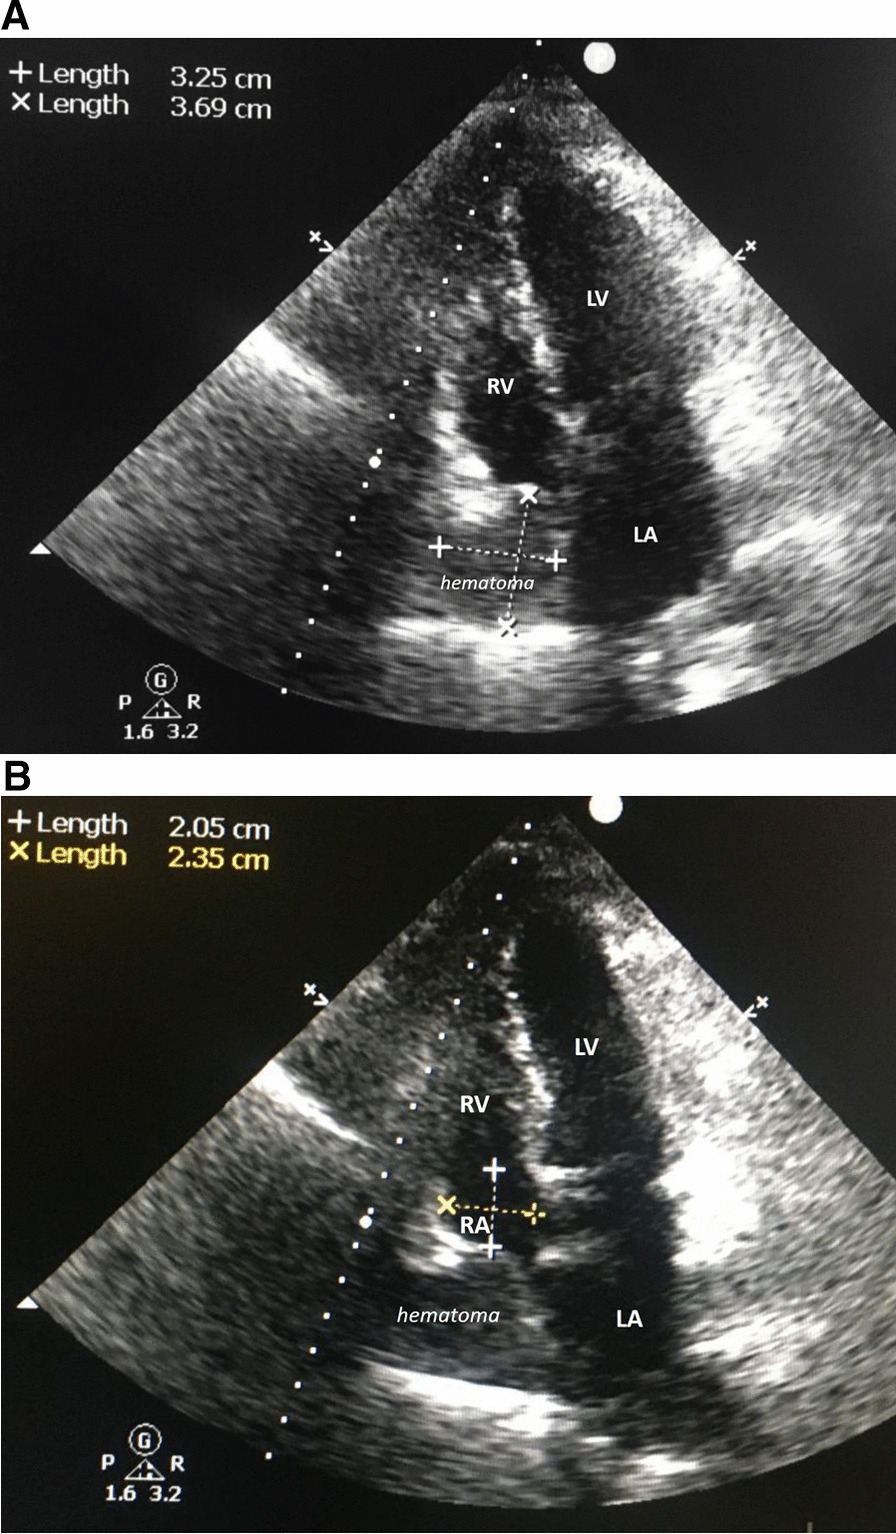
A Transthoracic echocardiography with apical four-chamber view, showing a round shaped 32 × 37 mm hematoma compressing the right atrium (systolic view). *LV* left ventricle, *RV* right ventricle, *LA* left atrium. B Transthoracic echocardiography with apical four-chamber view (after minor probe tilt) showing right atrial dimensions (20 × 23 mm) with compression by adjacent hematoma (systolic view). *LV* left ventricle, *RV* right ventricle, *LA* left atrium, *RA* right atrium (compressed)
